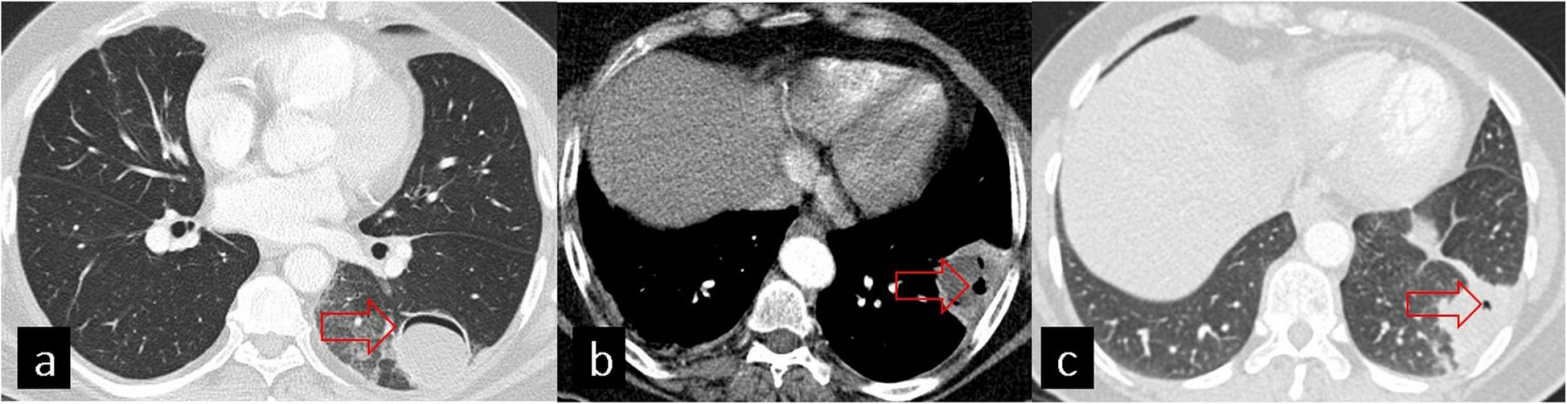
Imaging features of contained rupture. Axial CT images show the crescent sign (a, red arrow), inverse crescent sign (b, red arrow), and air bubble sign (c, red arrow)
